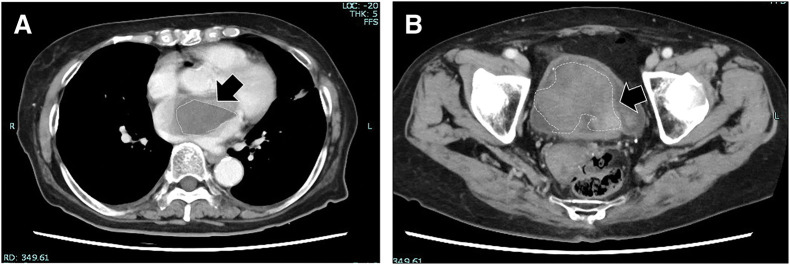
Preoperative imaging (A) Chest CT showing a 50-mm left atrial filling defect (arrow). (B) Pelvic CT showing an 8-cm hemorrhagic bladder tumor (arrowhead).
